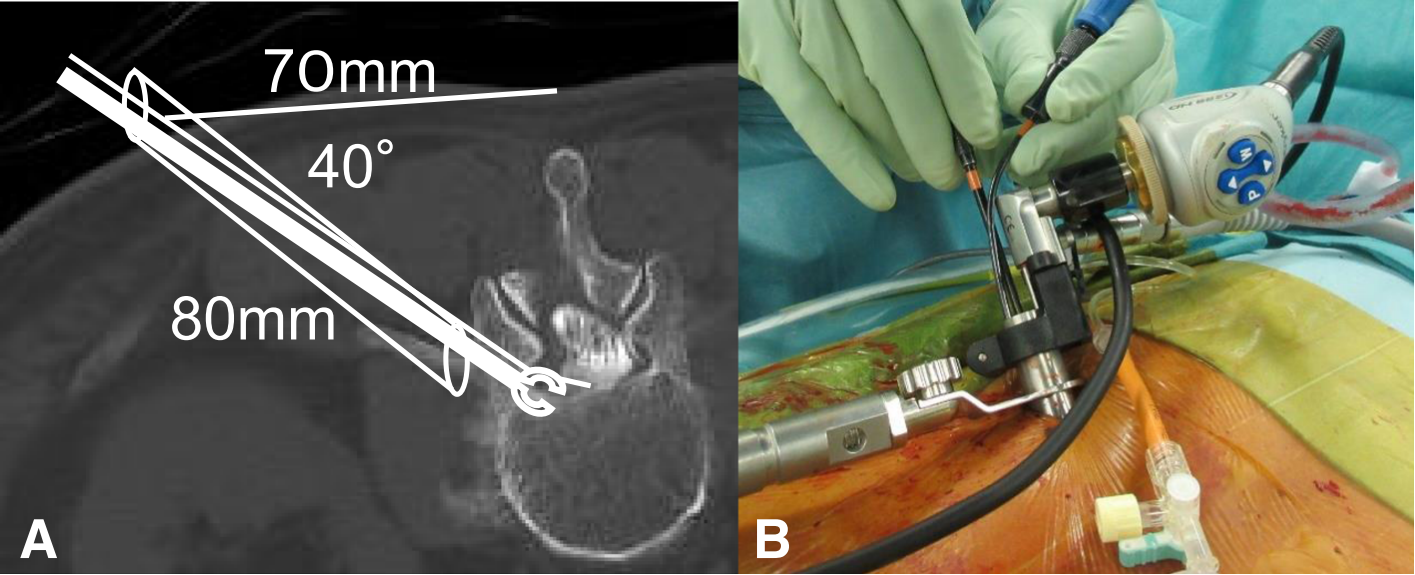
a Operation plan on computed tomography myelogram and b operation view
